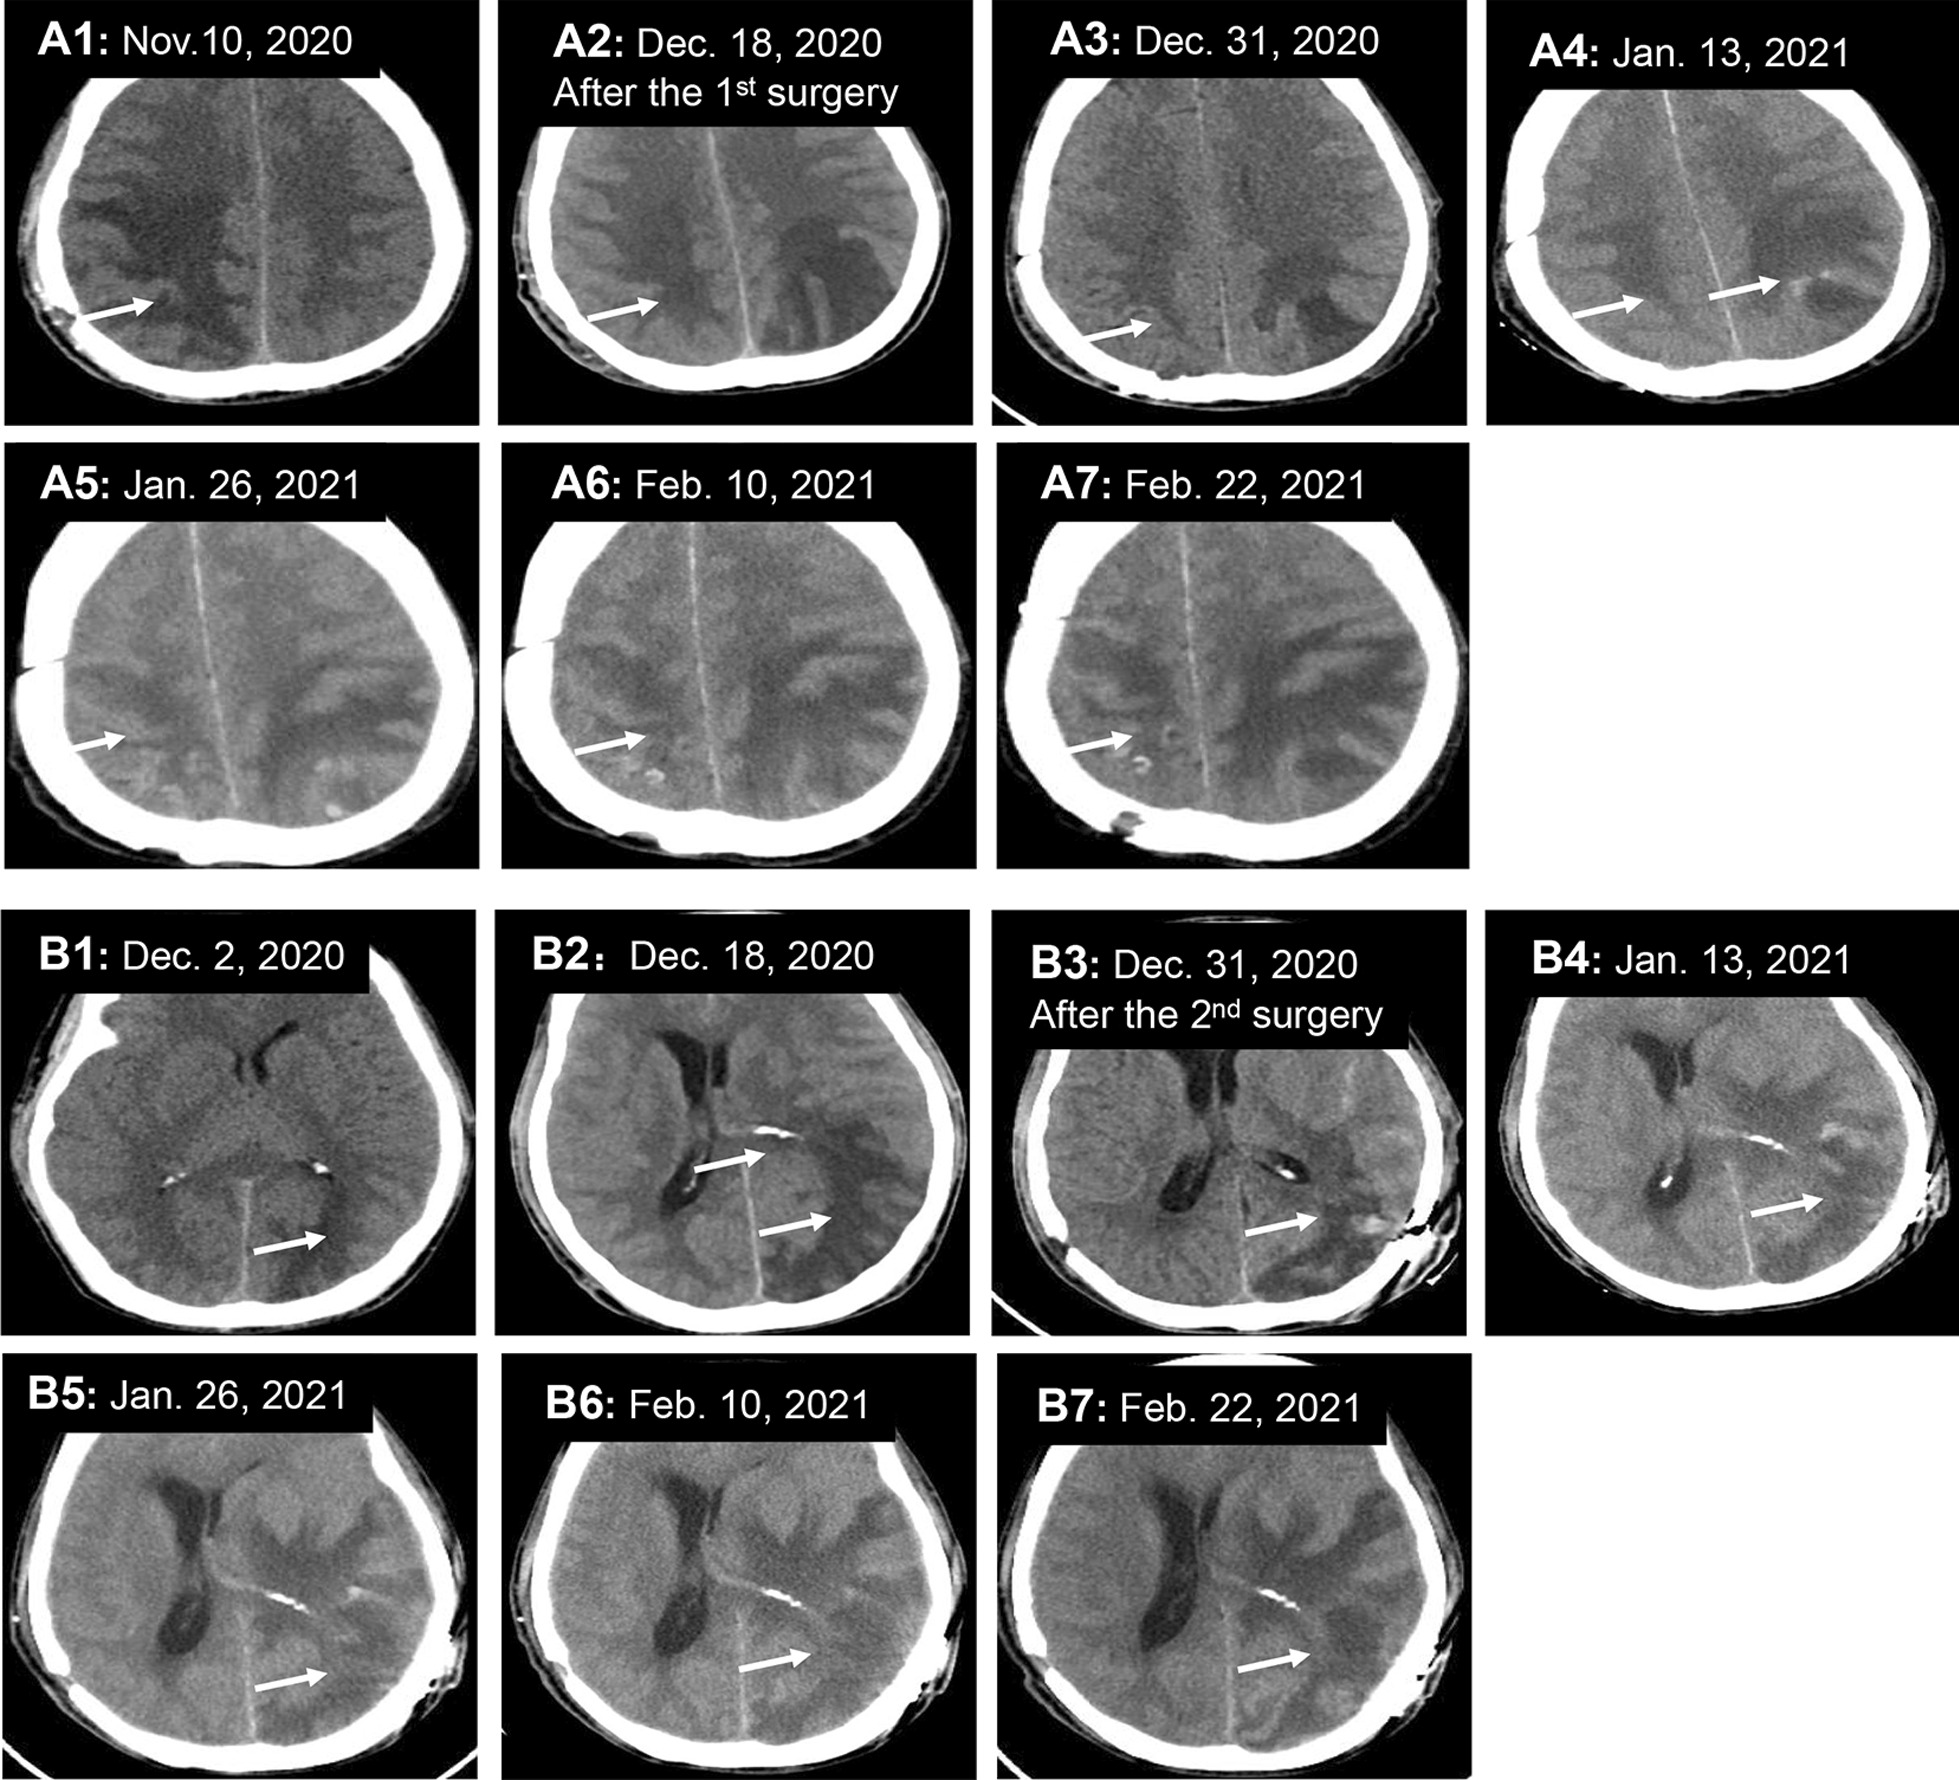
The dynamic changes of head CT. A The original lesion in the right parietal lobe was getting smaller; B a new lesion occurring in the left occipital lobe became worse
